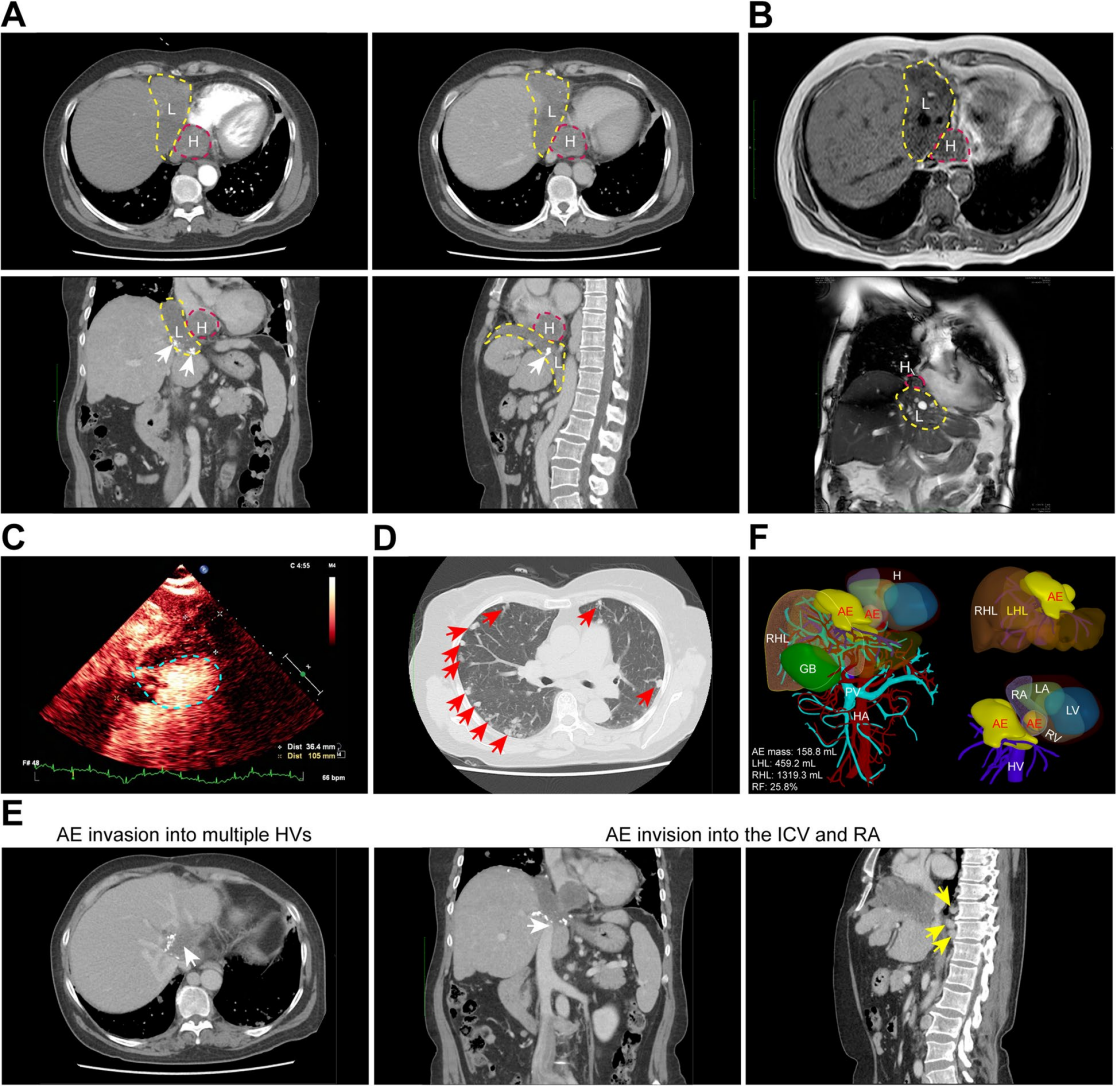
Preoperative imaging of the AE masses, indications for ex vivo liver resection and autotransplantation (ELRA), and volumetry of the patient’s liver. A Mixed density AE masses, delineated by yellow (L, liver) and red (H, heart) dashed circles, exhibit approximate dimensions of 7.11 × 6.02 cm, as discerned from the computed tomography (CT) and CT angiography (CTA). Situated around the right diaphragm, retrohepatic inferior vena cava (IVC), and the right atrium (RA), these unenhanced masses during the arterial phase (upper left) also displayed scattered calcification foci (white arrows; venous phase: upper right; coronal plane: bottom left; sagittal plane: bottom right). The venous phase further uncovered a mild dilation of the right branch of the portal vein (PV), disarray of the second hepatic portal, and an indistinct representation of the left hepatic vein (HV), and compression and narrowing of the retrohepatic IVC, attributed to the pressure from the masses. B The abdominal (above) and cardiac (below) magnetic resonance imaging (MRI) scans. The AE lesions, marked by yellow (L, liver) and red (H, heart) dashed circles, were identifiable in the left lobe, caudate lobe, and tail lobe of the liver. With indistinct boundaries, these lesions measured approximately 7.96 × 3.45 cm at their largest extent. The left branch of the PV exhibited partial obscurity, and the HVs are thickened (below); concurrently, a mass was discerned near the RA. C A cystic-solid mixed mass observed in the cardiac ultrasound angiography, located below the diaphragm near the RA side wall at the hepatic portal, and this mass was suspectedly located within the RA. D CT angiography (CTA) shows symmetrical thorax with diffused nodules and blocky lesions in both lungs (indicated by red arrows). The largest measured about 1.86 × 1.60 cm. Slight atelectasis was noted in the right middle lobe's pericardial outer edge and the left upper lobe's lingual segment. Multiple mediastinal and hilar lymph nodes with increased density suggest homologous pulmonary AE lesions. E Abdominal CT scans indicate a severe invasion of the AE lesion into multiple HVs, IVC, and the RA (white arrows), with numerous circulating lateral branches forming around the blood vessels of the vertebrae (yellow arrows). F The three-dimensional (3D) reconstruction of the patient’s liver, heart, AE lesions, and major vessels, along with the volumetry of the liver and the lesions (Available at: https://yun.gradient3d.com/scene/case3d.html?ValidCode=230709&SID=b94e05f8-4e36-4773-8c3c-29fa31696896). The residual liver post-resection (right hemiliver, RHL) is highlighted in yellow. AE, alveolar echinococcosis; GB, gall bladder; H, heart; HA, hepatic artery; HV, hepatic vein; LA, left atrium; LHL, left hemiliver; LV, left ventricle; PV, portal vein; RA, right atrium; RF, resection fraction; RHL, right hemiliver; RV, right ventricle. For comprehensive images of the CT and MRI scans, kindly refer to the accompanying videos
